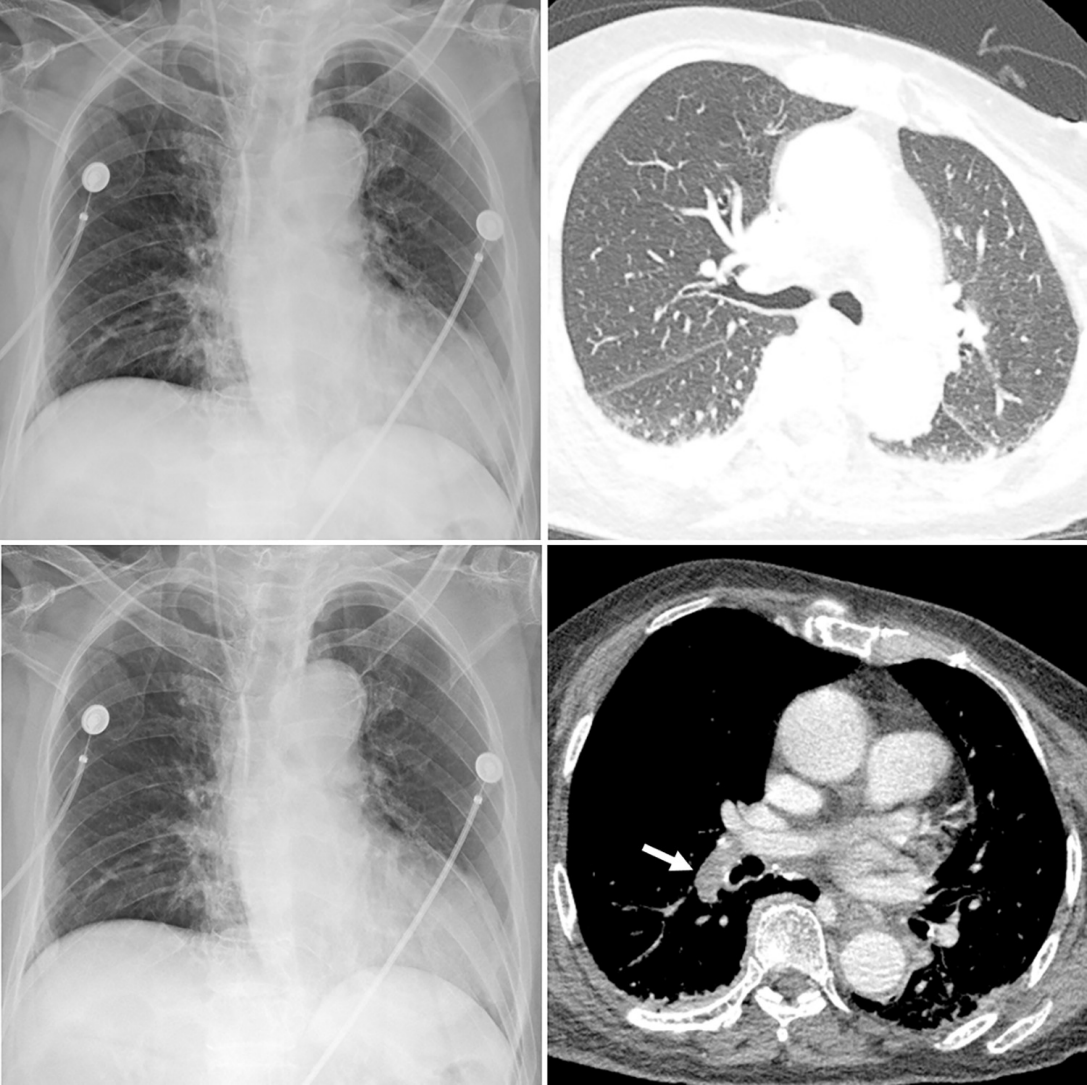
Representative case without COVID-19 pneumonia. A CXR (A) and corresponding chest CT (B) of a patient with fever and dyspnea but negative RT-PCR result for COVID-19 show no pulmonary abnormality suggestive of pneumonia. The CAD system did not detect any abnormalities in the CXR and the probability score was 13% (C). In the reader-alone interpretation, four thoracic radiologists and four non-radiologist physicians misclassified the CXR as having findings of pneumonia. In the CAD-assisted interpretation, only one thoracic radiologist and two non-radiologist physicians made false-positive classification of the CXR. Mediastinal window CT image (D) show pulmonary embolism in the right descending pulmonary artery (arrow), presumed cause of patients’ symptom.
